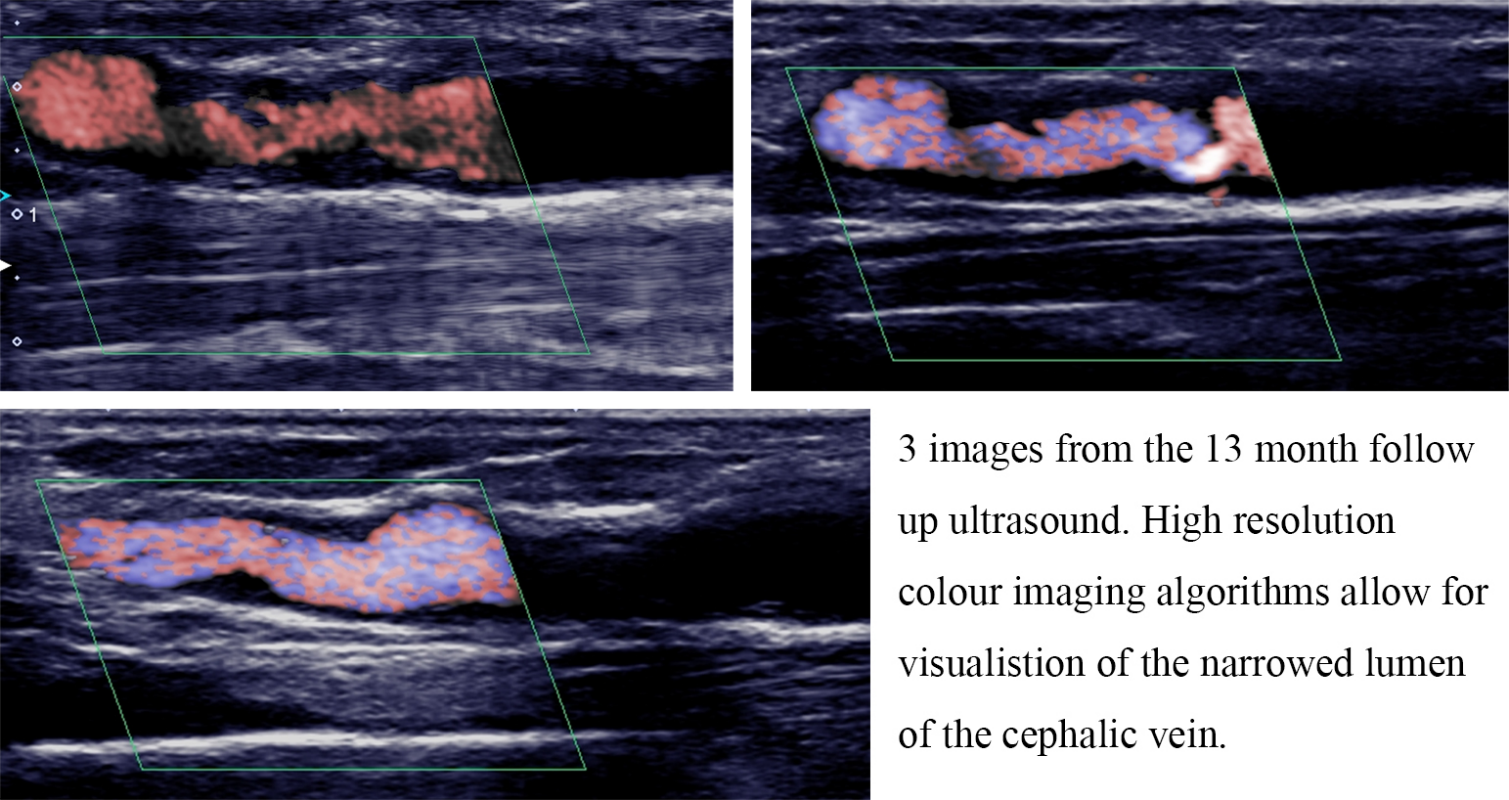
Ultrasound images showing development of neointimal hyperplasia
